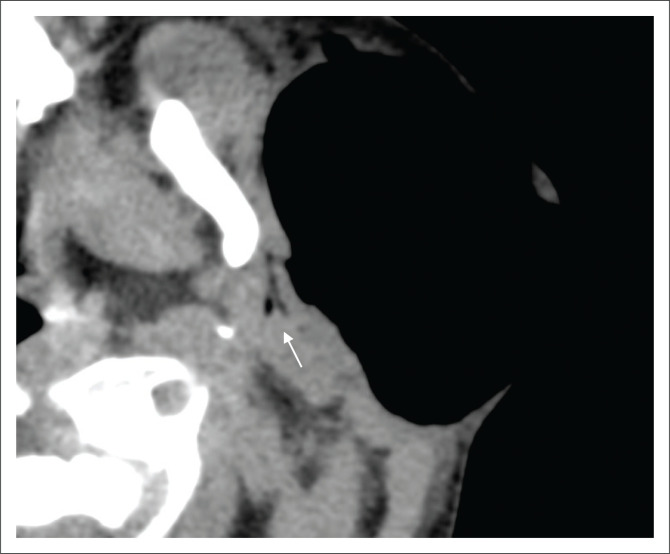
Magnified axial non-contrasted computed tomography with soft tissue window again demonstrates the gas-filled branching parotid ductal pattern (white arrow). The left parotid gland appears otherwise morphologically normal, with no features of inflammation.
